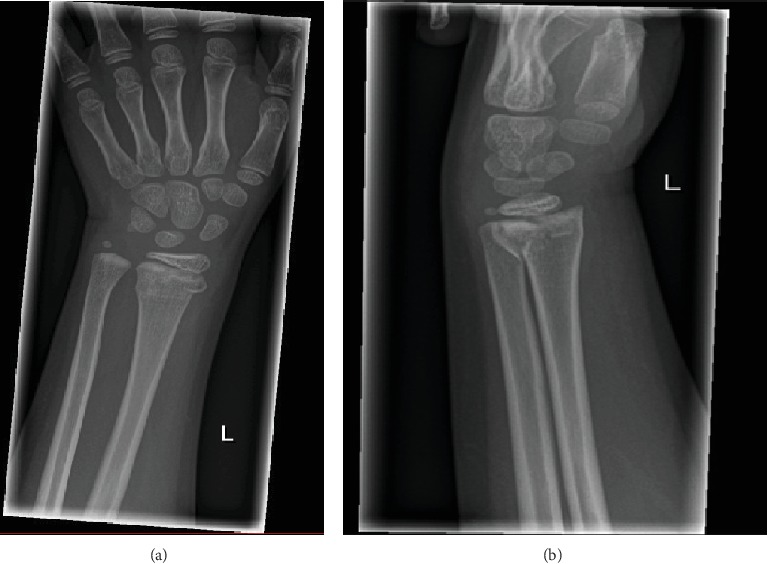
(a, b) Anteroposterior and lateral radiographs of the left wrist distal forearm fracture showing a Salter-Harris type 2 fracture of the distal radius and a greenstick fracture of the distal ulna.
